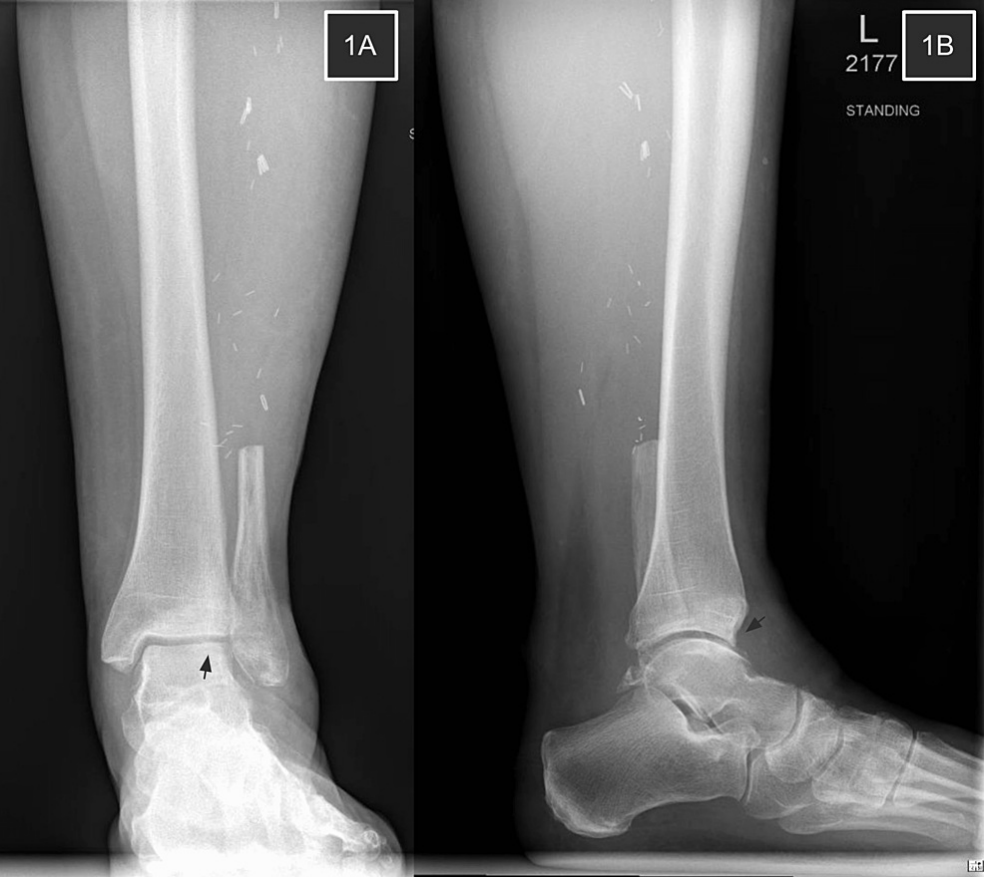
Ankle radiographs four months post-FVFG 1A shows irregularity of the lateral shoulder of the talar dome without valgus deformity of the ankle joint. 1B reveals a small anterior distal tibial osteophyte without talar subluxation in the sagittal plane.
